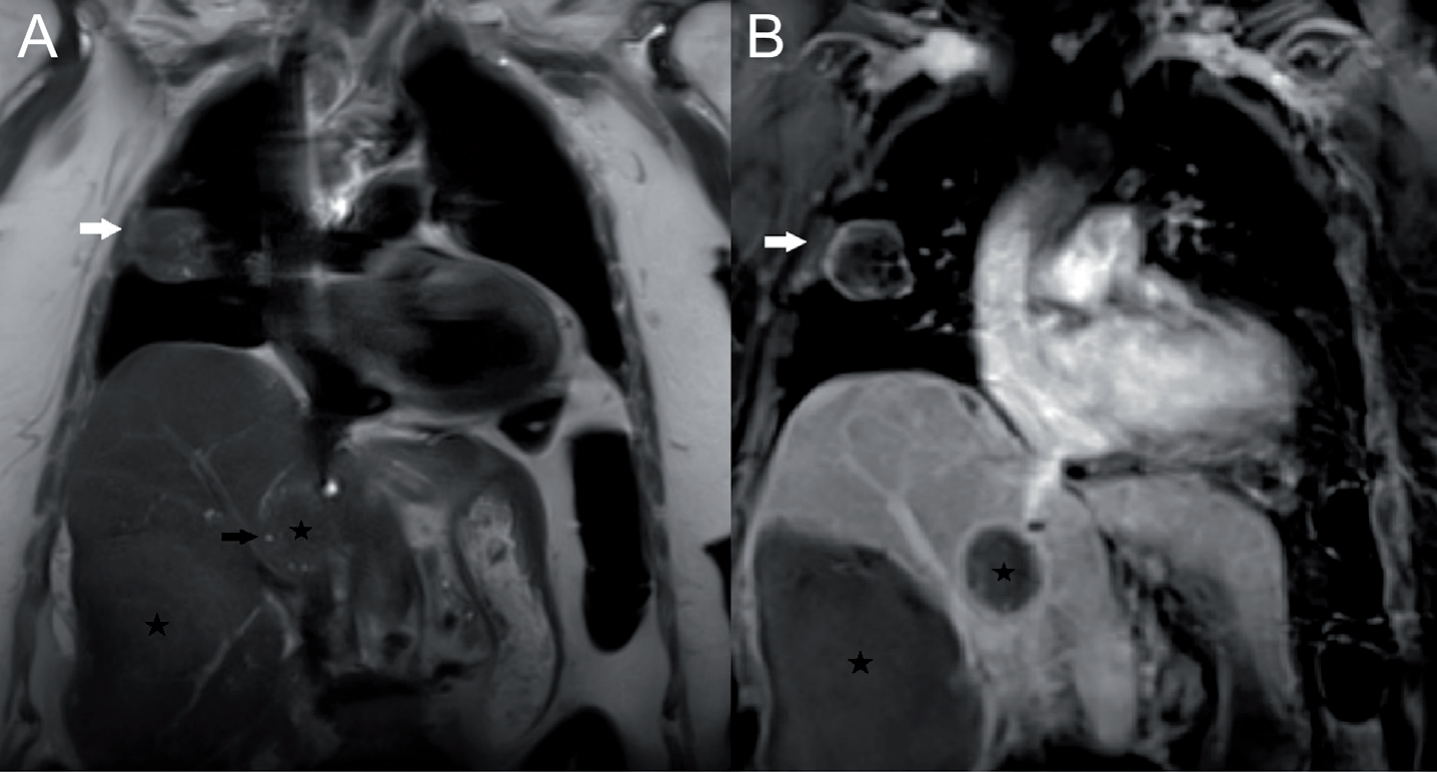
Coronal T2-weighted magnetic resonance image (A) and coronal T1-weighted contrast-enhanced image (B) show alveolar echinococcosis lesions with microcysts and solid components in the liver (black stars). The black arrow indicates the microcystic content in the lesions observed in the liver. The patient also had another alveolar echinococcosis lesion invading the pleura in the right lung (white arrow).
